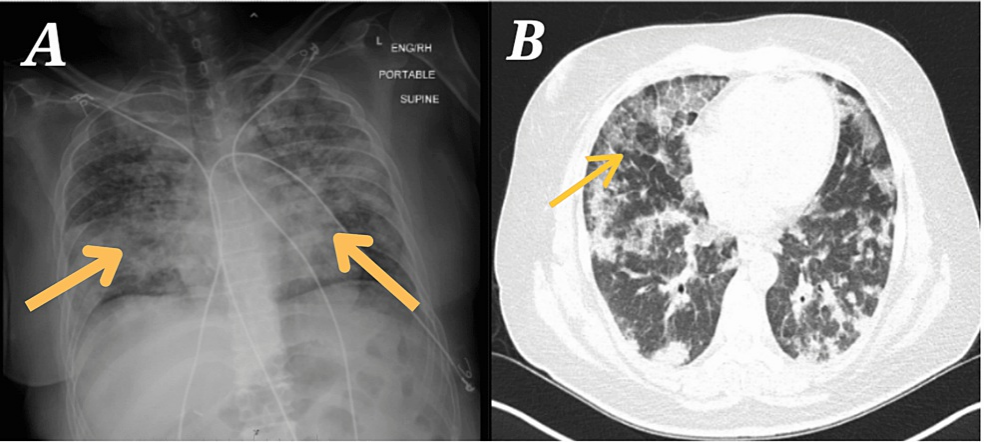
Chest X-ray and CT images of the patient's lungs A: Chest X-ray showing hazy opacities; B: CT scan showing crazy paving pattern
